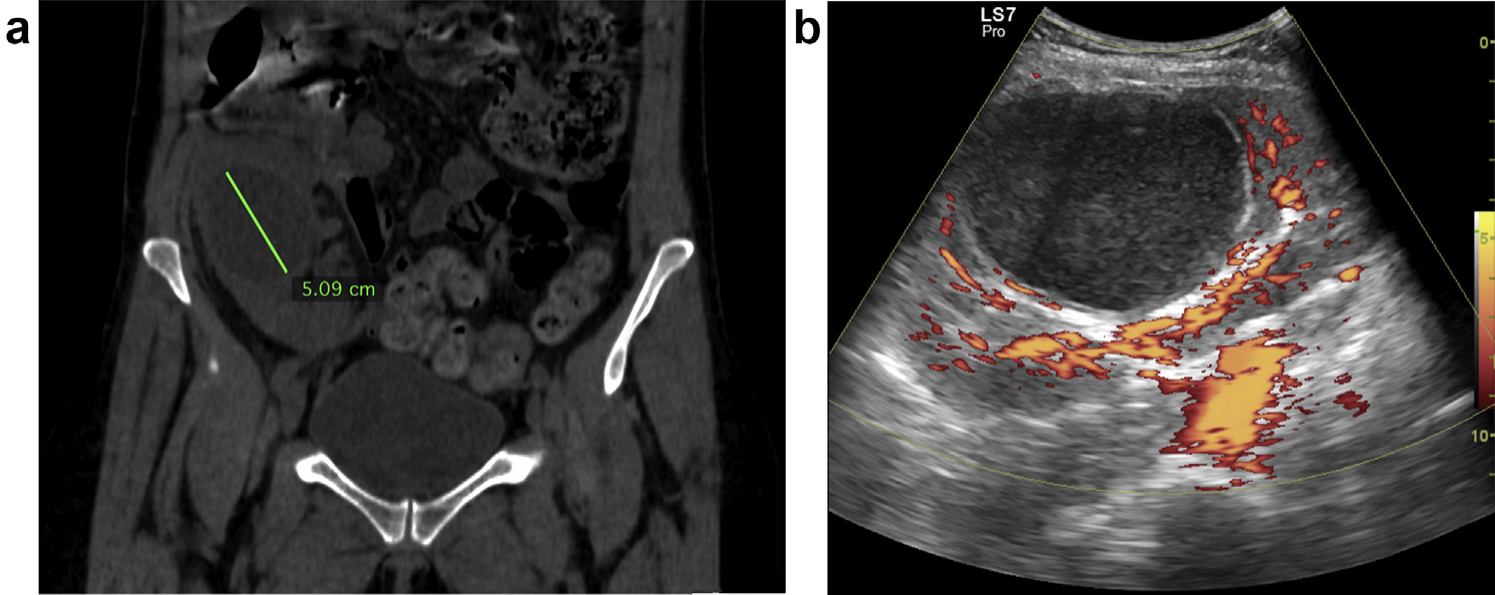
(a) Computed tomography image of the transplant recipient at 1 year after transplantation, showing the initially unroofed cyst, which regained full size due to allograft encapsulation, and (b) an ultrasound of the kidney allograft with signs of simple cyst infection.
